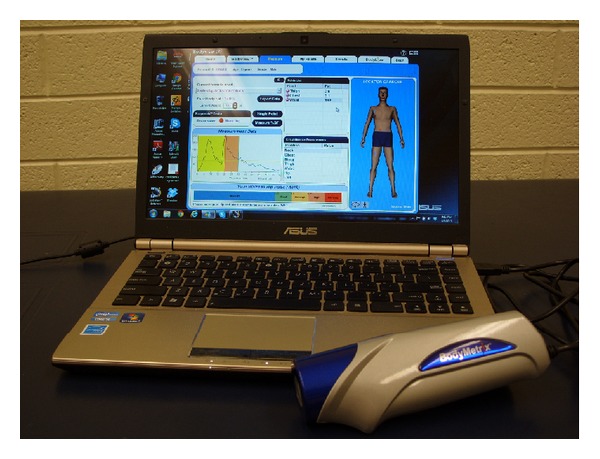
BodyMetrix ultrasound with body composition software.
